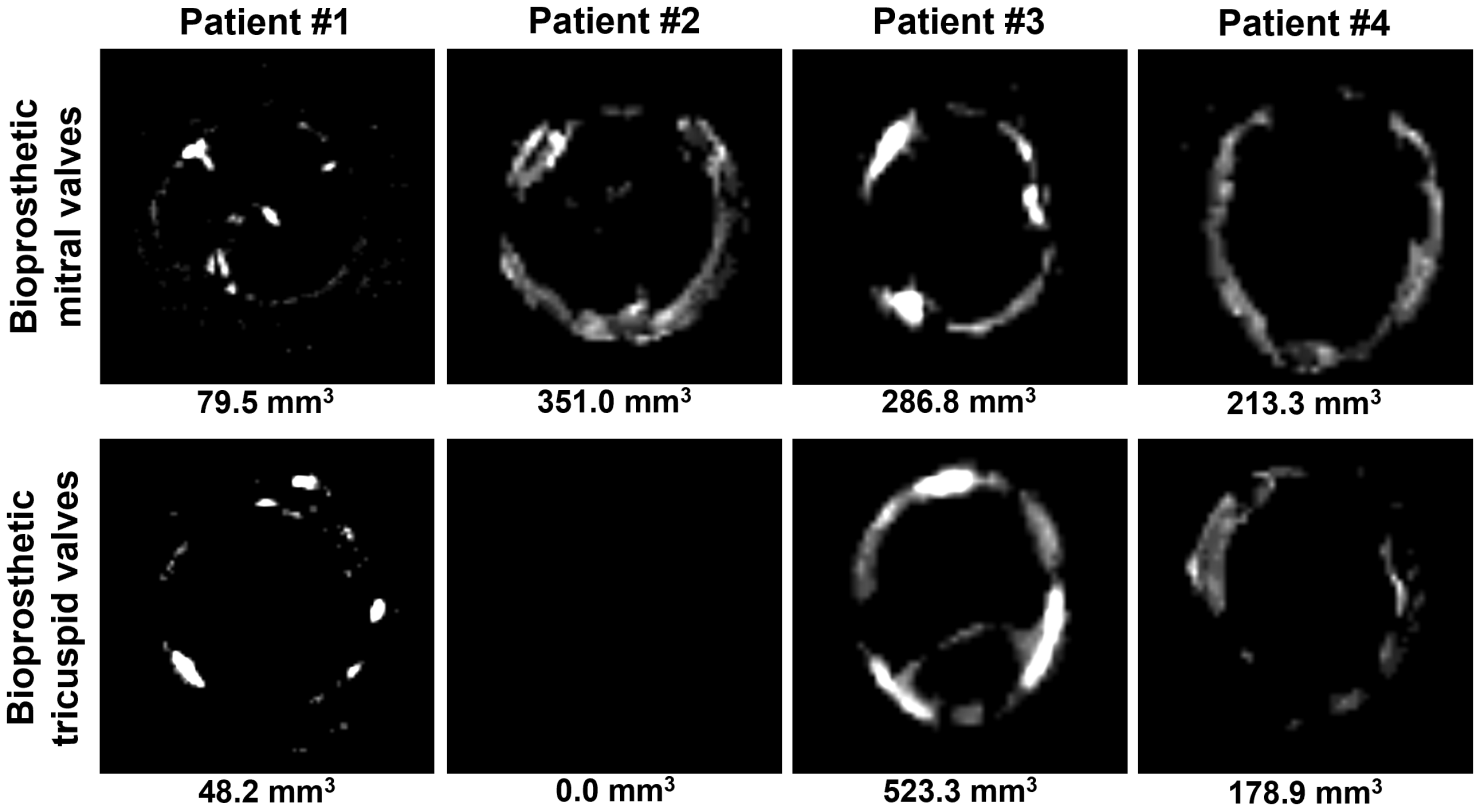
Visualization of calcium deposits in bioprosthetic mitral valves (BMVs, top) and bioprosthetic tricuspid valves (BTVs, bottom) by an averaged 2D projection of multislice computed tomography images. Calcification volume is indicated below each image.
